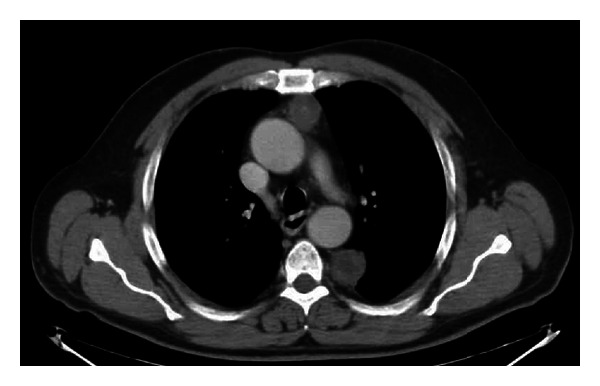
Computerized tomography (CT) scan showing a left posterior mediastinal schwannoma associated with an anterior parathyroid adenoma.
